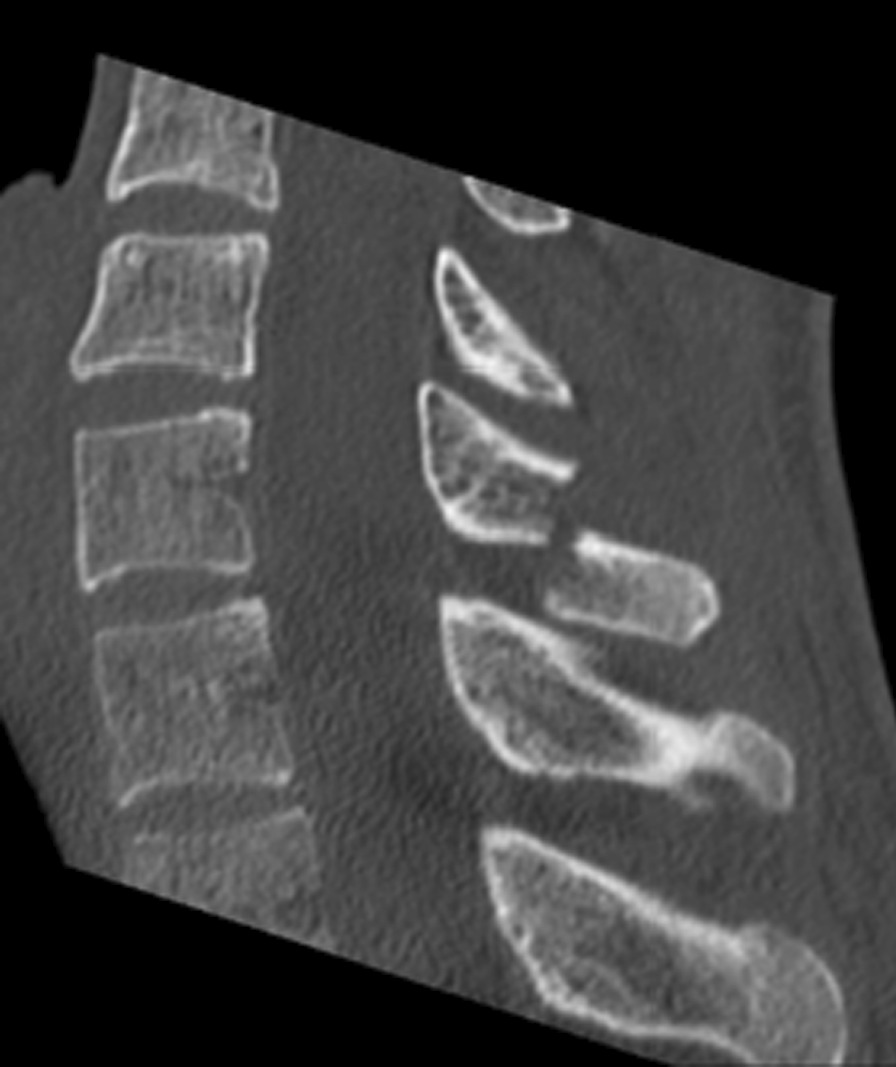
Lateral computed tomography (CT) scan, X-ray, CT and magnetic resonance imaging taken from patient folder with the patient’s consent
